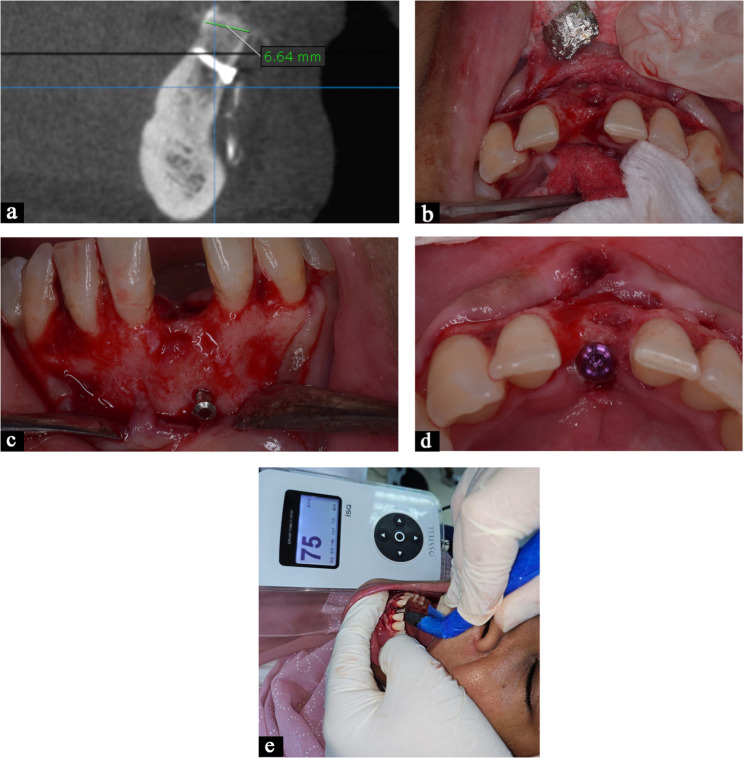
a Postperative CBCT from DVR module b Flap design for implant placement occlusal view c Flap design for implant placement frontal view d Implant placement e Osstell reading for primary stability
